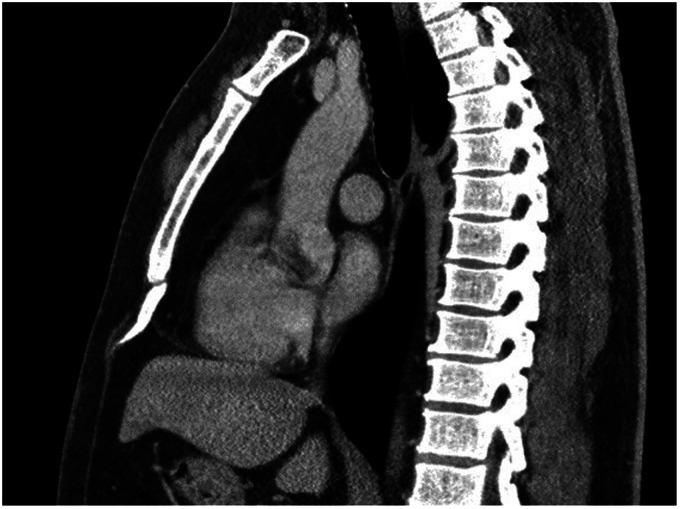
CT image (sagittal plane) showing the xiphoid process bending forwards
